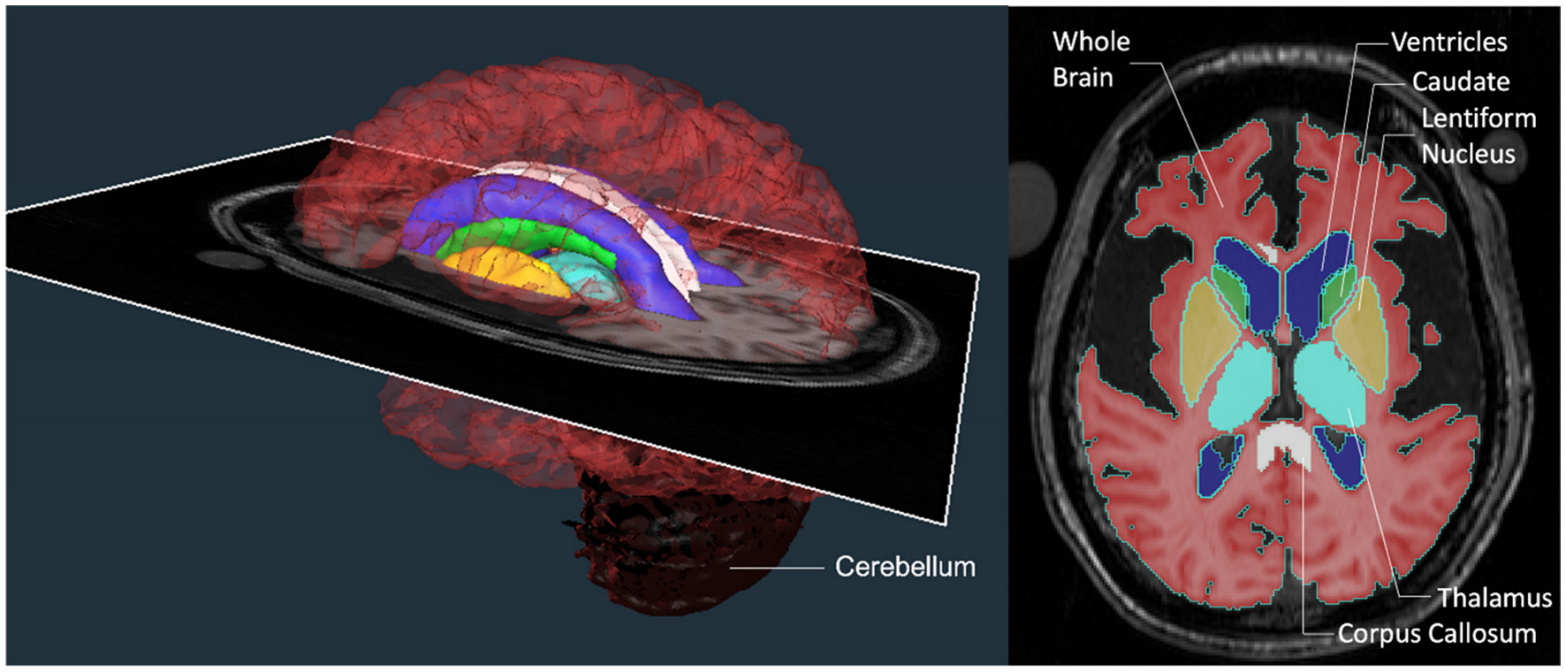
Brain region segmentations. A color-coded 3D rendering of a patient's brain with an axial MRI slice transecting it at the level of the image shown on the right. The axial slice on the right demonstrates the regions of interest (ROIs) that were segmented at each level on the scan to generate the 3D structures for comparison. The ROIs consisted of structures that were large (whole brain without ventricles, ventricles, and cerebellum) and small (lentiform nucleus, thalamus, corpus callosum, and caudate).
